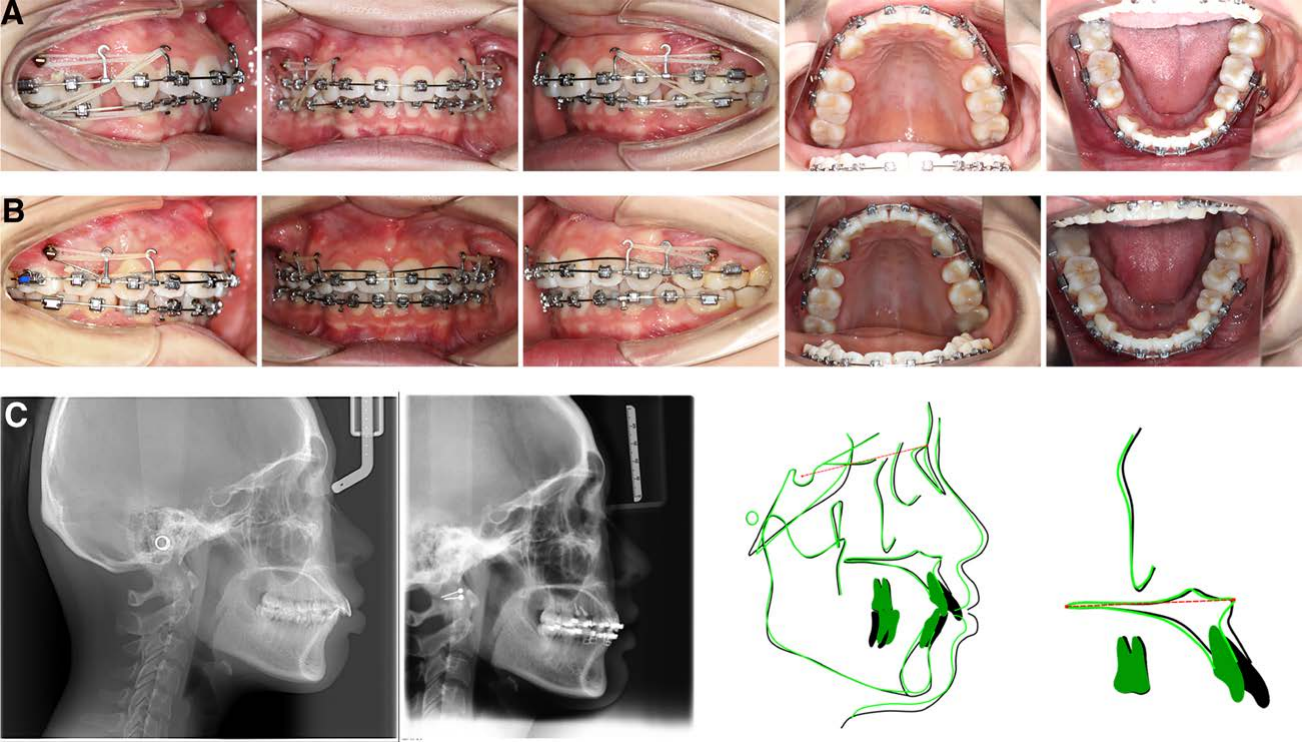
The intraoral features and cephalometric superimposition of patient 2. (A) The intraoral features of patient 2 before incisors intrusion. (B) The intraoral features of patient 2 after incisors intrusion. (C) pretreatment and post-treatment cephalometric radiographs and superimposed tracings of patient 2: black line, pretreatment; green line, after incisor intrusion. The upper incisor was apparently intruded.
